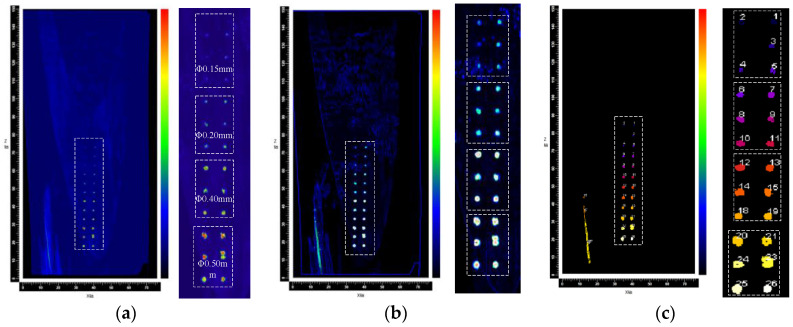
Ultrasonic C-scan images for defects with a diameter of 0.15 mm. (a) Original ultrasonic C-scan image. (b) Identification of defect contours for contrast. (c) Defect identification calibration.
